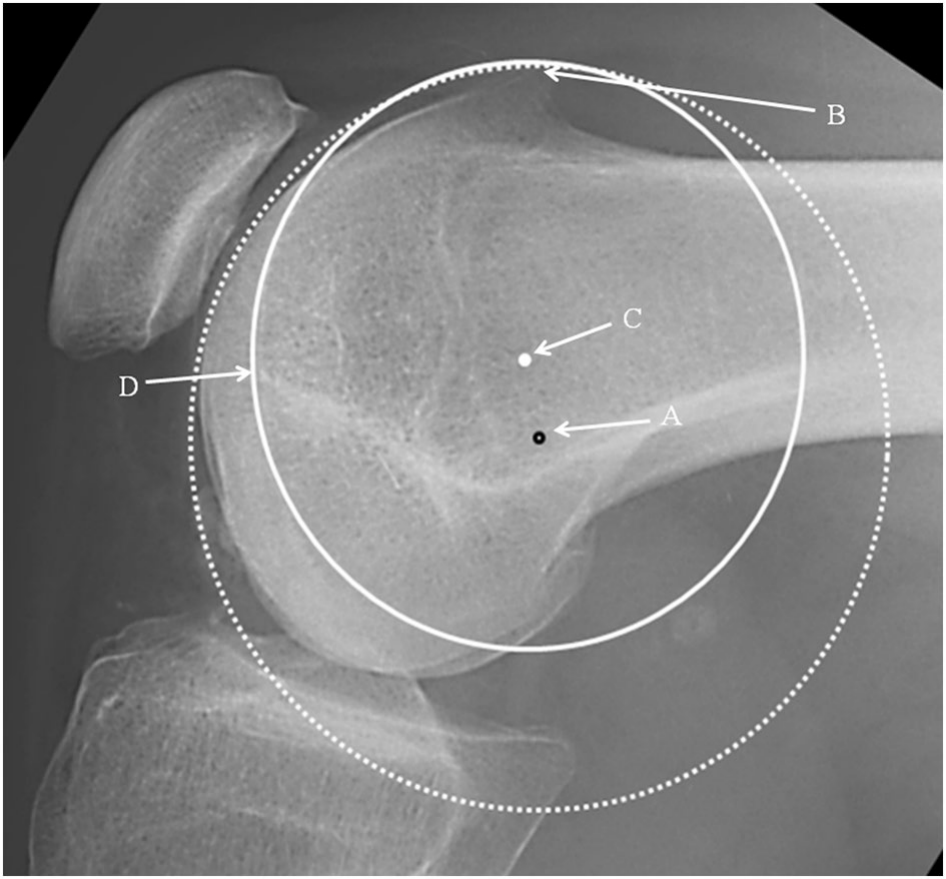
Lateral radiograph of the knee showing: Schöttle’s point: black rimmed spot (A). Isometric point: white spot (C). Dotted circle: centred on Schöttle’s point with the radius to the highest point of the boss (B). Complete circle: centred on the isometric point. Note that the dysplastic groove is not an arc of this circle. Point D is the distal end of the notch
